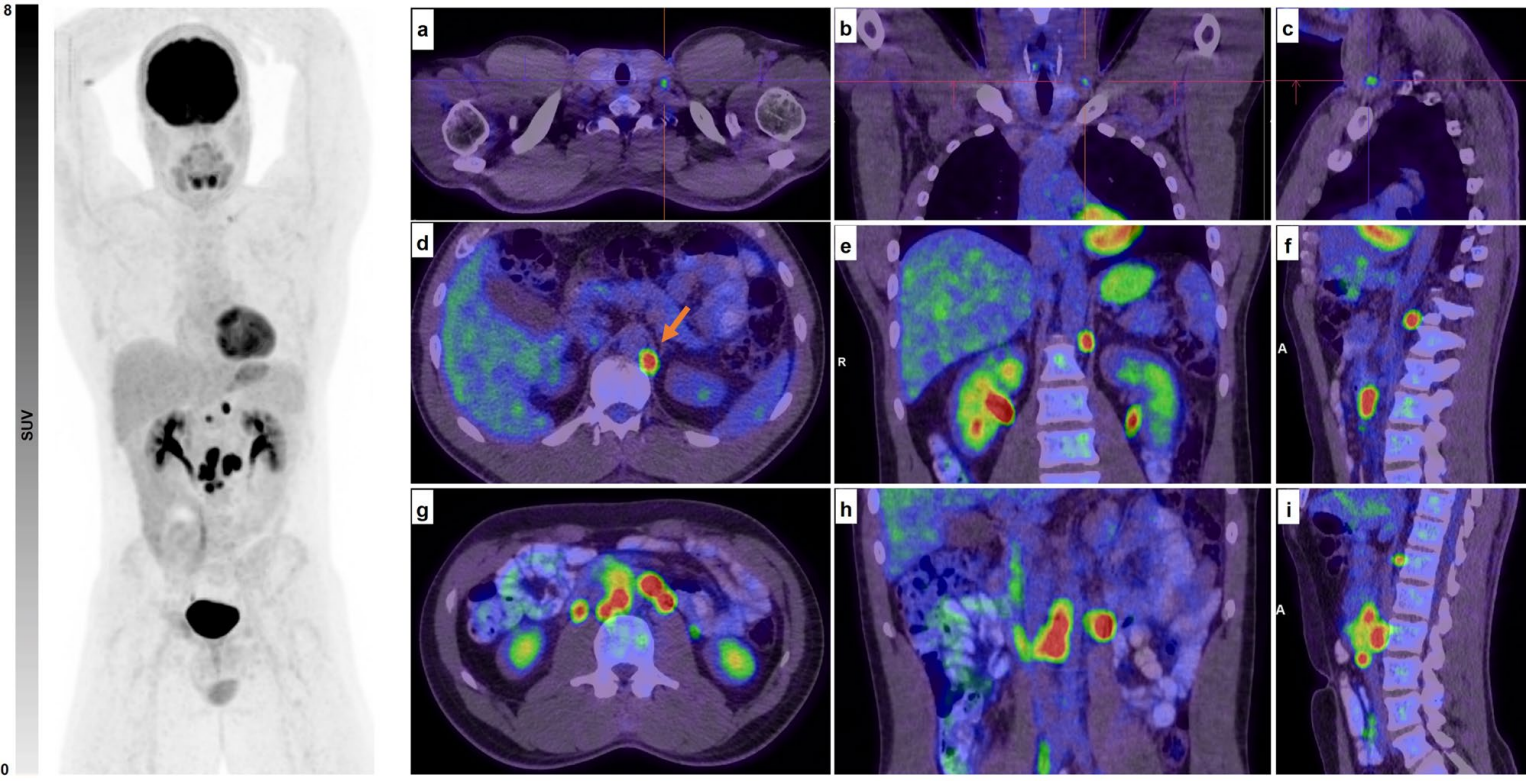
A 32-year-old male with non-seminomatous testicular cancer, initially treated with orchidectomy, presented with suspicious para-aortic lymph nodes on CT and underwent an FDG PET scan. The images reveal a subcentimetre left supraclavicular lymph node (SUVmax 3.5; images a-c, crosshairs), bilateral intensely FDG-avid retrocrural lymph nodes (SUVmax 8 on the right and 12 on the left; images d-f, red arrow), and bilateral para-aortic lymphadenopathy extending from the axial level of L1 to L2/L3 on the right (SUVmax 21) and from the axial level of L1/L2 to L2/L3 on the left (SUVmax 25; images g-I). A subsequent biopsy of the left supraclavicular node confirmed metastatic involvement. Retroperitoneal lymph node dissection also confirmed multifocal retroperitoneal nodal metastases
